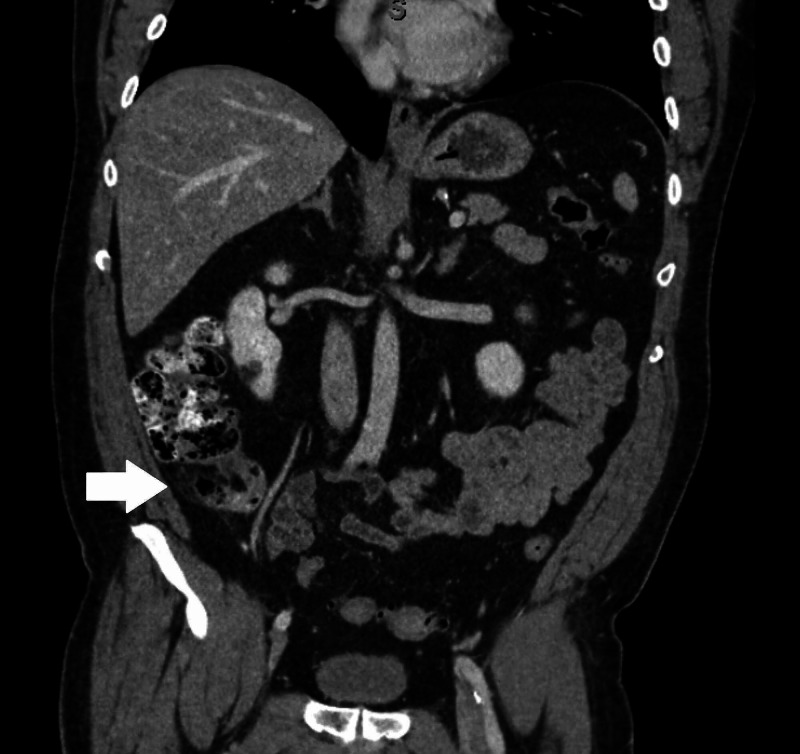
Coronal view of CT showing dilated and inflamed appendix.
